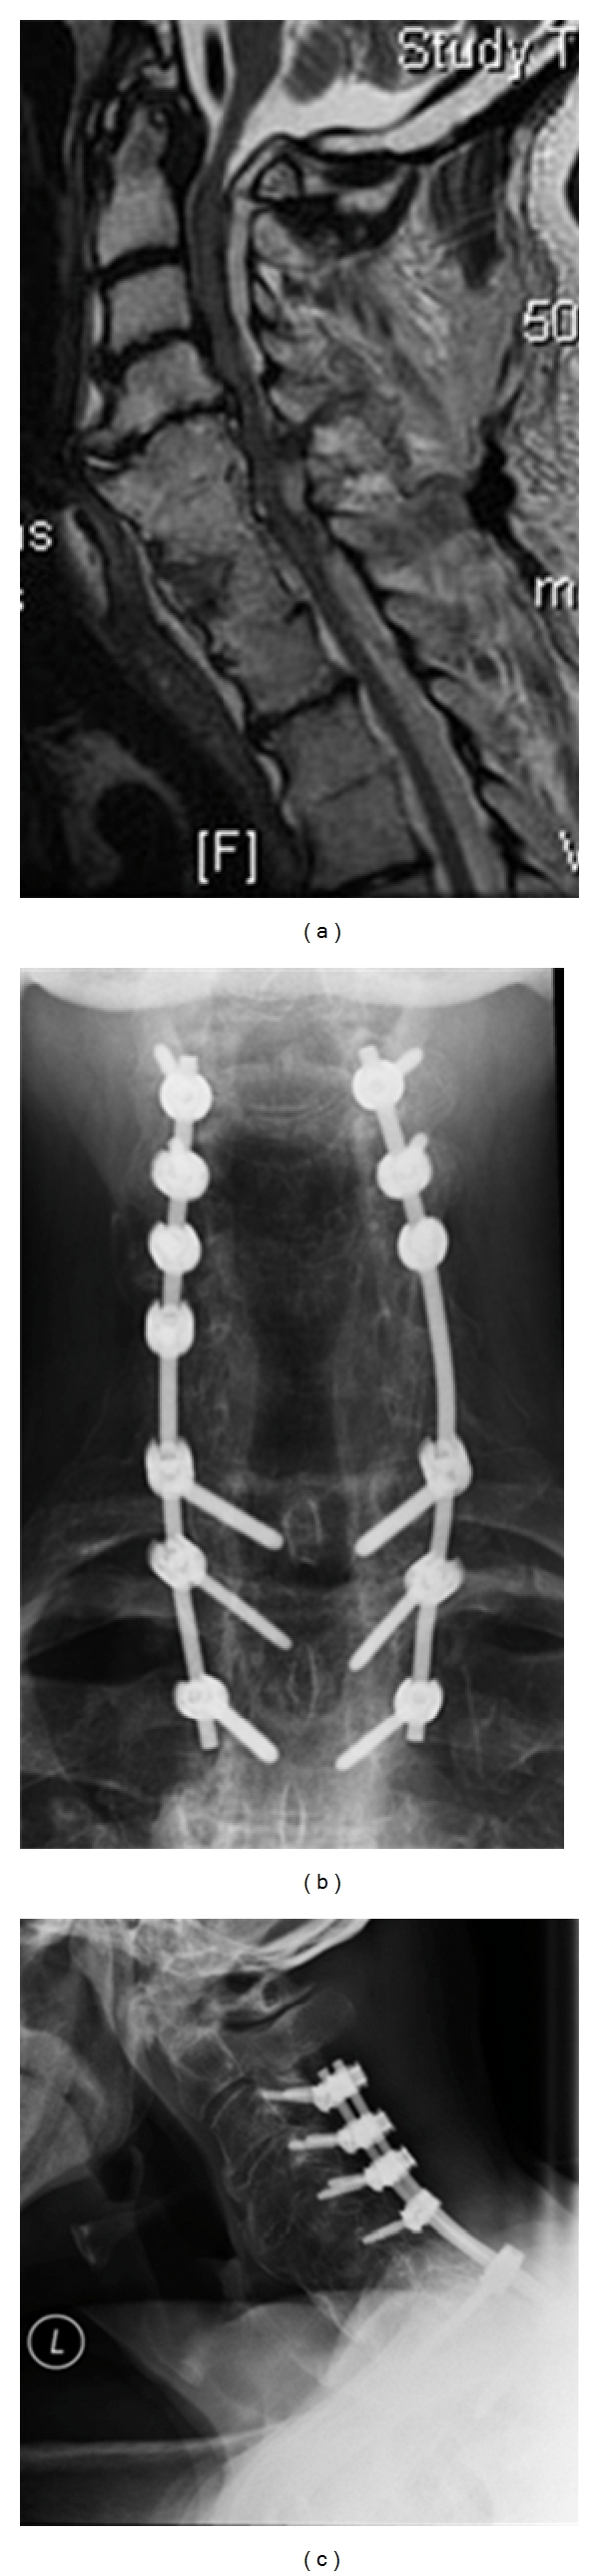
(a) Sagittal MRI of a patient with severe multilevel spondylosis and stenosis. (b-c) Anteroposterior (b) and lateral (c) radiographs of same patient after multilevel laminectomy and fusion. Note that distal fixation was achieved with pedicle screws which have increased pullout resistance compared with lateral mass fixation.
